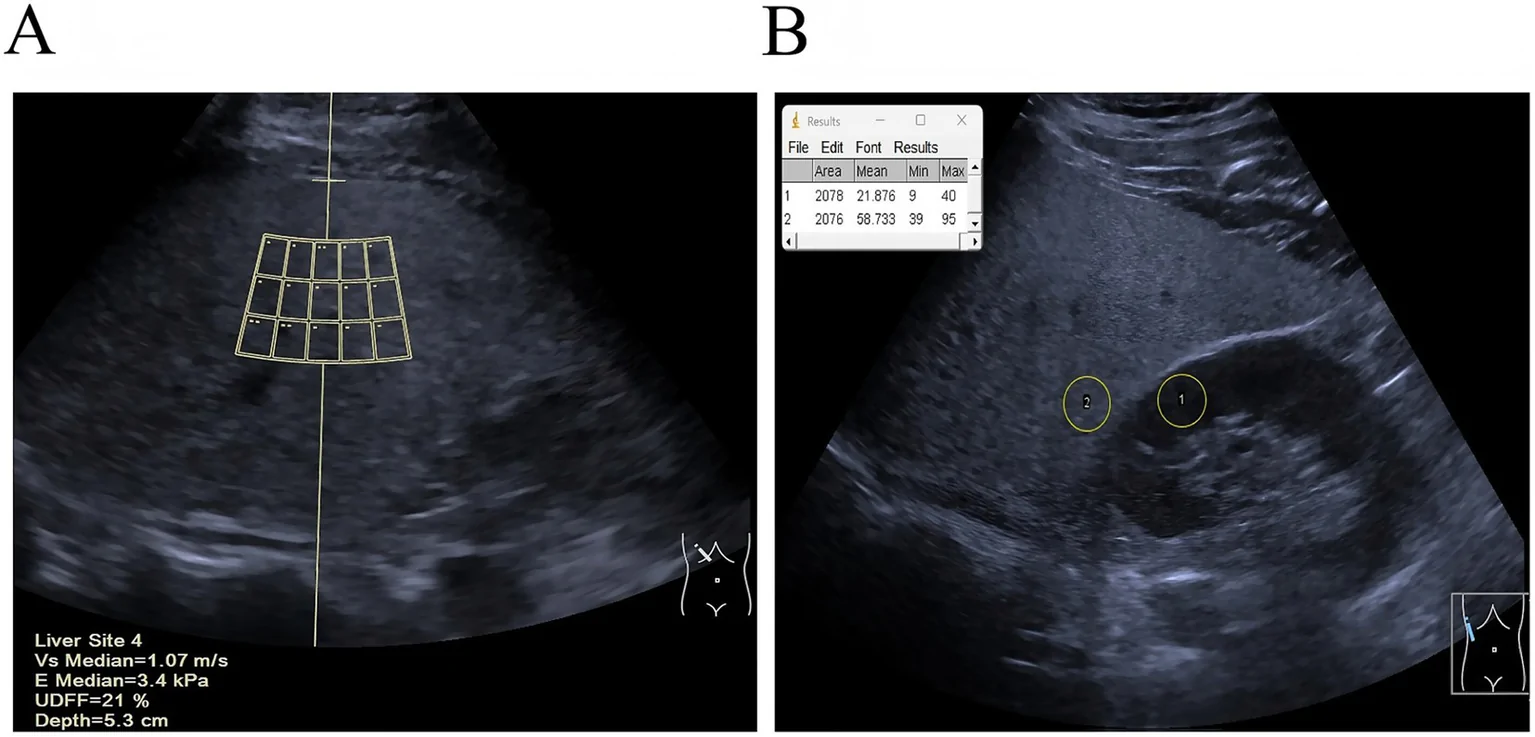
Ultrasound assessment of hepatic steatosis. (A) Prospective UDFF acquisition using the dedicated DAX transducer on the ACUSON Sequoia system. (B) Offline ImageJ-based HRI measurement from an archived liver-kidney B-mode image acquired using the 5C1 transducer. Equal-sized circular ROIs were placed in the liver parenchyma and right renal cortex at comparable acoustic depths. HRI, hepatorenal index; ROI, region of interest; UDFF, ultrasound-derived fat fraction.
